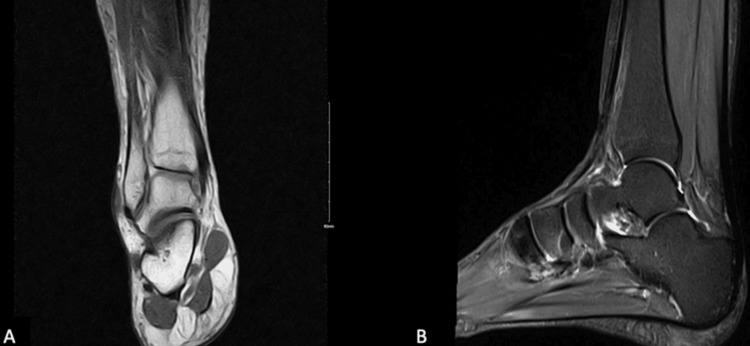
(A, B) MRI images for a 41-year-old male with chronic ankle pain and instability. Radiological diagnosis showed preservation of normal anatomy.
